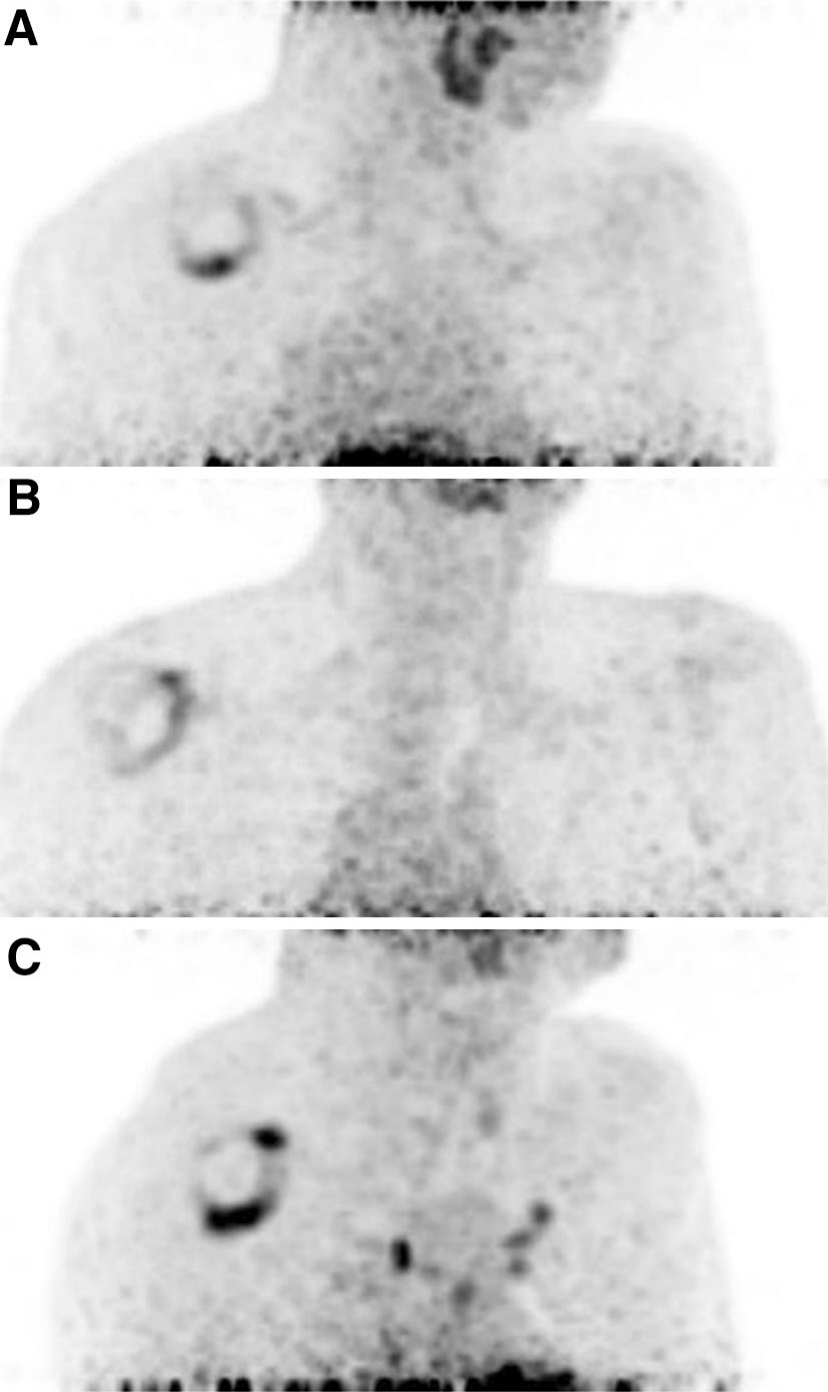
Three recognizable patterns of increased ^18^F-FDG uptake. a AR dominant type. b RI dominant type. c Both RI and AR dominant type
